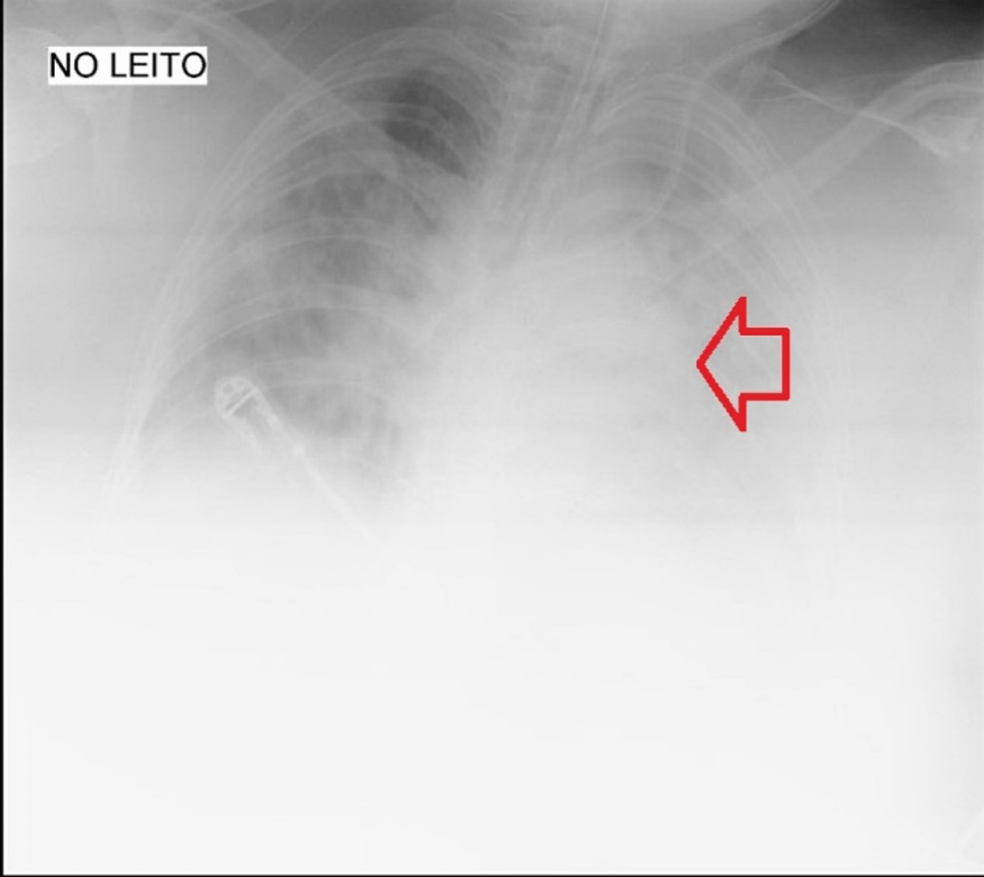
Chest radiography of the day before the start of corticosteroid therapy The red arrow shows opacities
